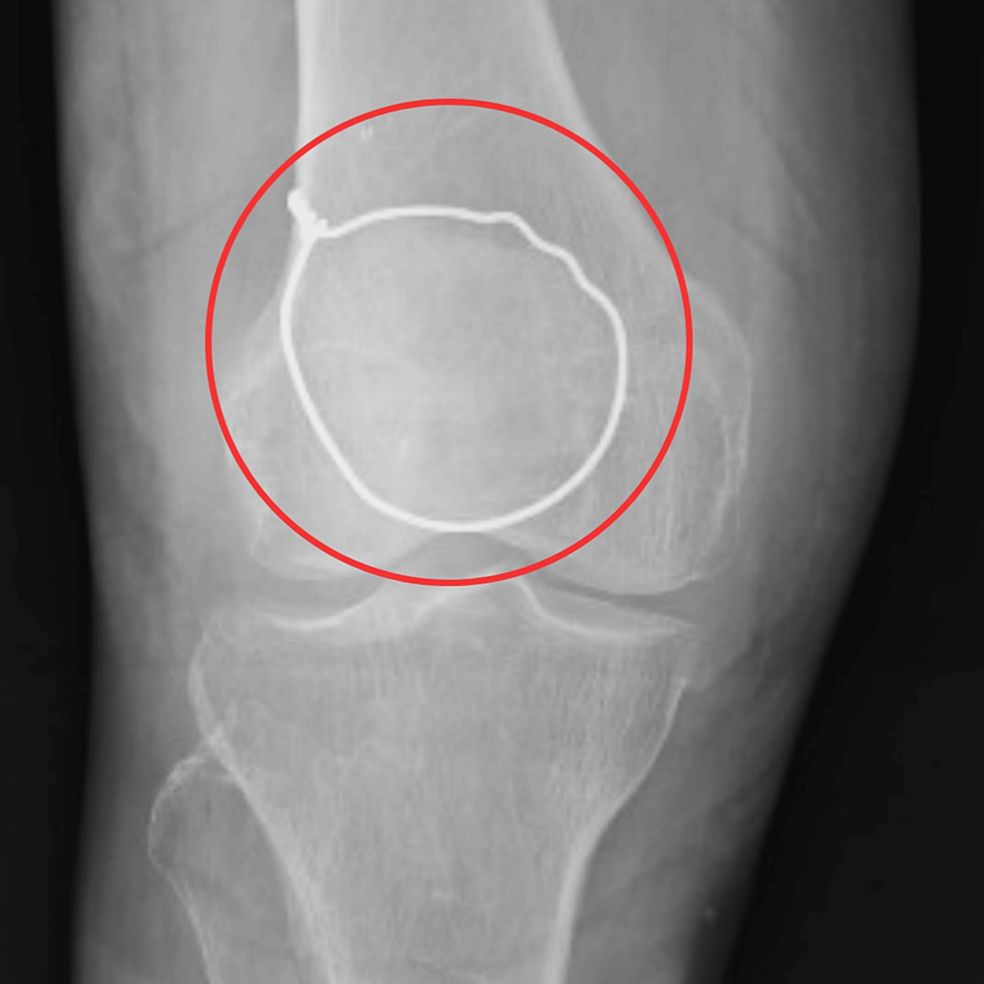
X-ray of the post-operative patella The red circle shows the fixation of fractured patellar fragments with Kirschner wire.
